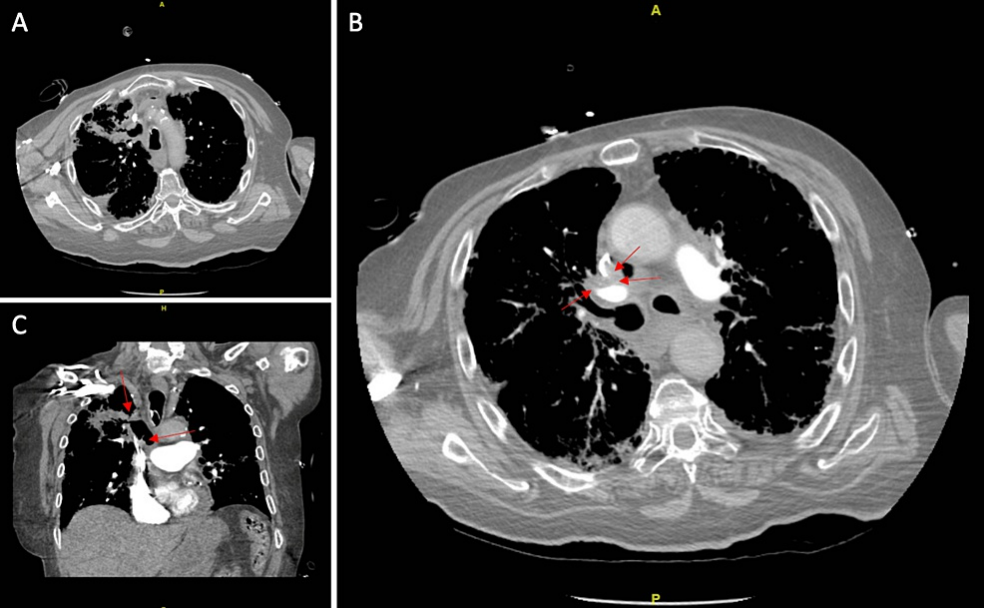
Contrast-enhanced axial CT images of the chest, timed with a CT pulmonary angiogram protocol. (A) Axial view of chest at the level of the aortic arch demonstrating cavitary mass with communication of the cavitary space with a right upper lobe bronchus. (B) Axial view of the chest at the level of the main pulmonary artery (inferior to A). Arrows demarcate soft tissue component of the mass which demonstrates intraluminal erosion into the superior vena cava. (C) Coronal view of the chest incorporating axial images A and B. Red arrows depict communication of the cavitary component of the mass to the bronchus and erosion of the mass into the SVC. Constellation of findings compatible with bronchovenous fistula from lung carcinoma.
